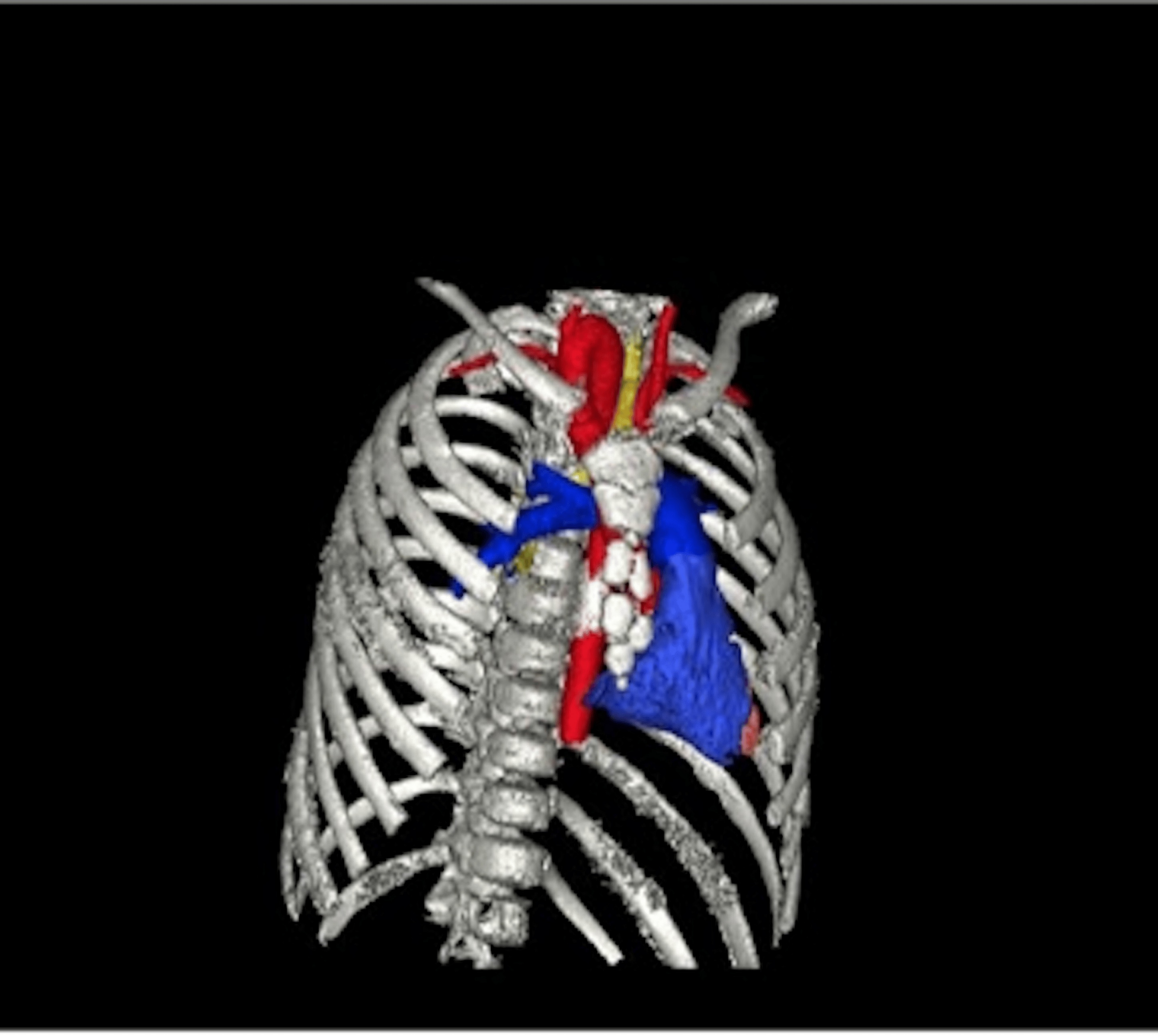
Volume-rendered 3D model from chest CTA showing the spatial orientation of the abnormalities within the rib cage in the anterior view CTA, CT angiography
